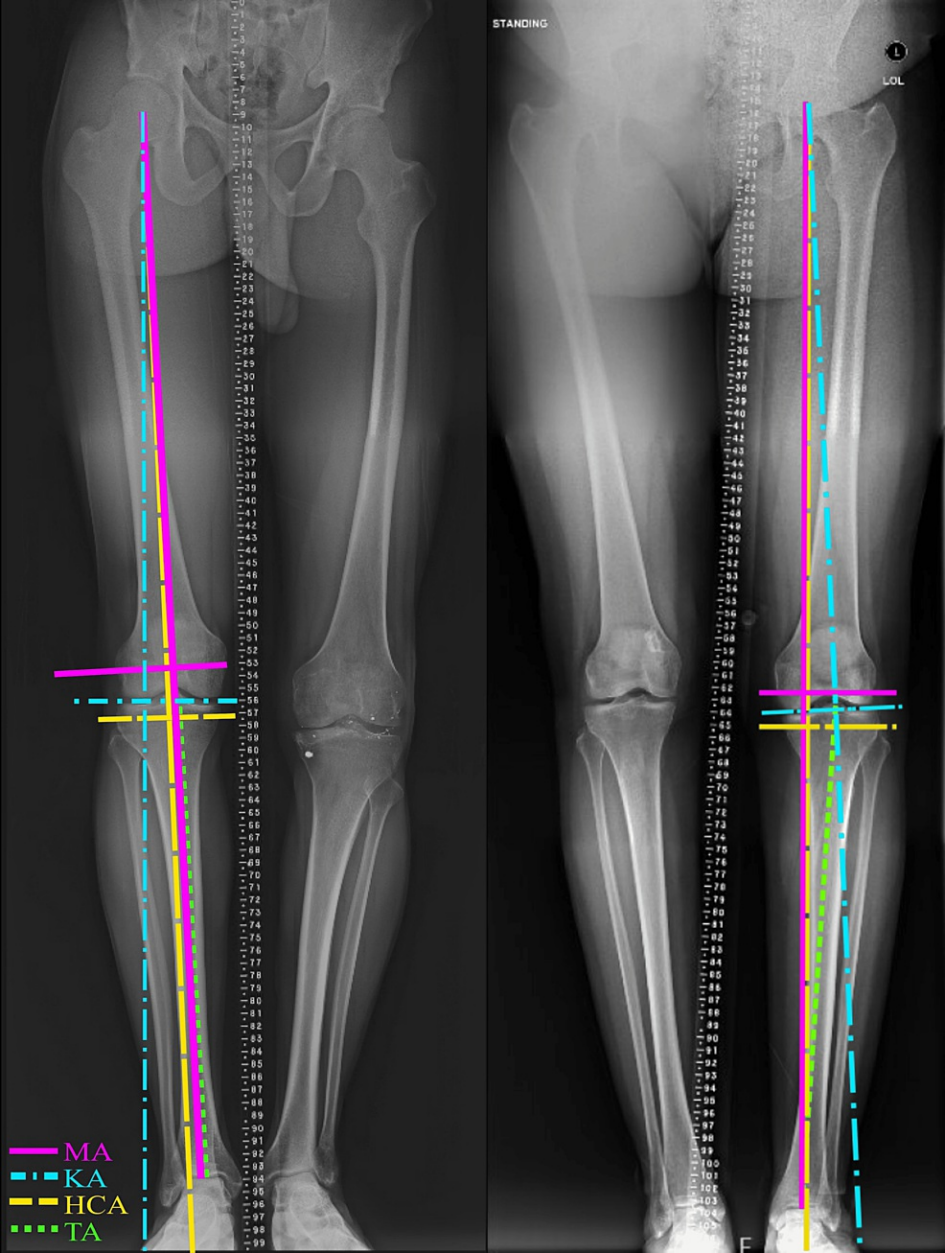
Two full bone-length standing hip-to-ankle digital radiographs of two different patients that were analyzed, depicting the mechanical, kinematic, hip-to-calcaneus, and tibial-ankle axes. MA=mechanical axis and its perpendicular joint-line; KA=kinematic axis and its perpendicular joint-line; HCA=hip-to-calcaneus axis and its perpendicular joint-line; TA=tibial-ankle axis.
